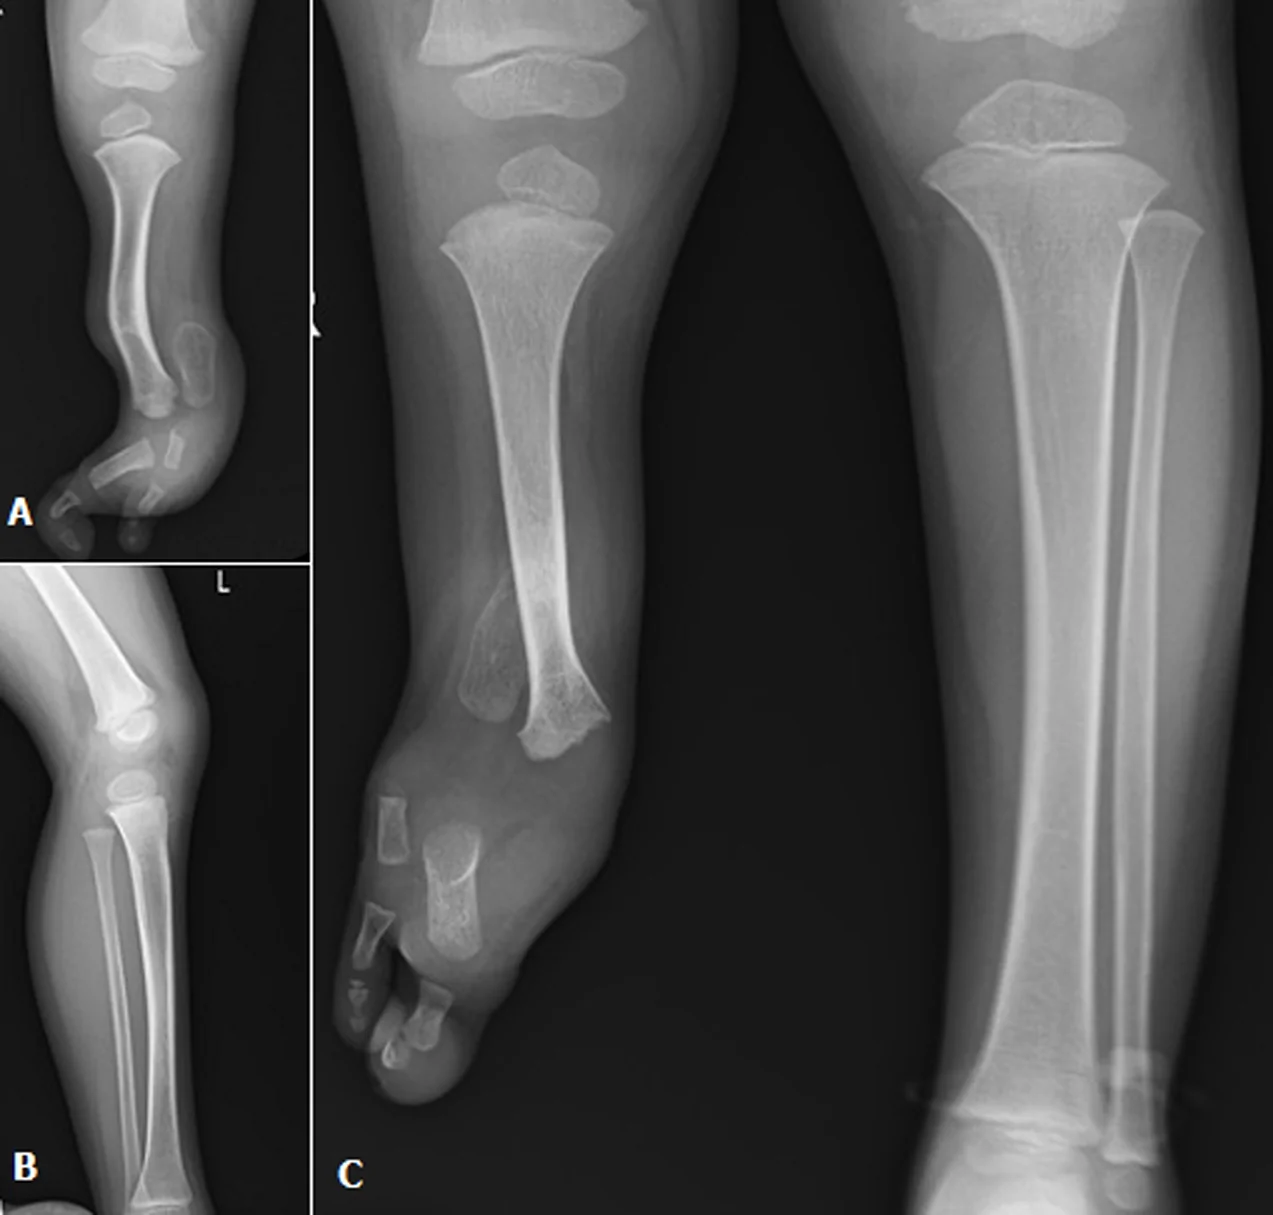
radiographs of the lower limbs showing: A, B) anteroposterior and lateral views of the right leg demonstrating complete absence of fibula, anterior bowing of the tibia, and oligosyndactyly of the right foot; C) comparative view of both lower limbs showing a normal left leg and shortened, deformed right leg consistent with FATCO syndrome
